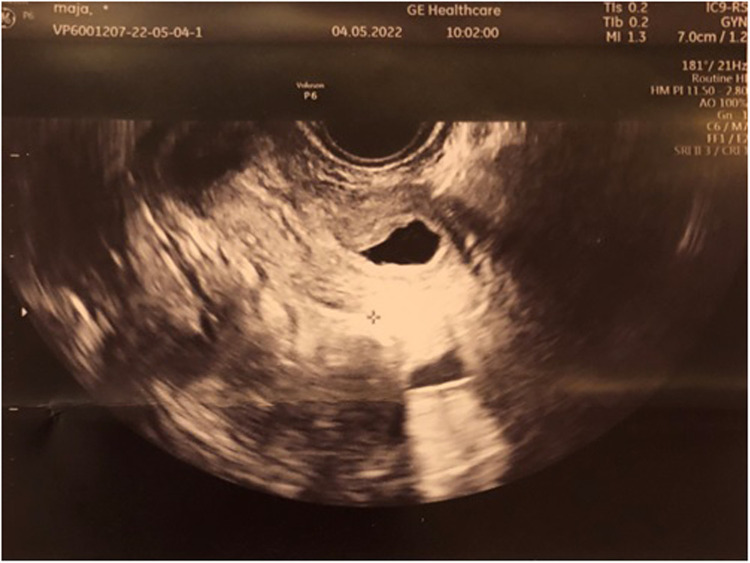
Intraoperative transvaginal sonography shows the resolution of hematocolpometra.
